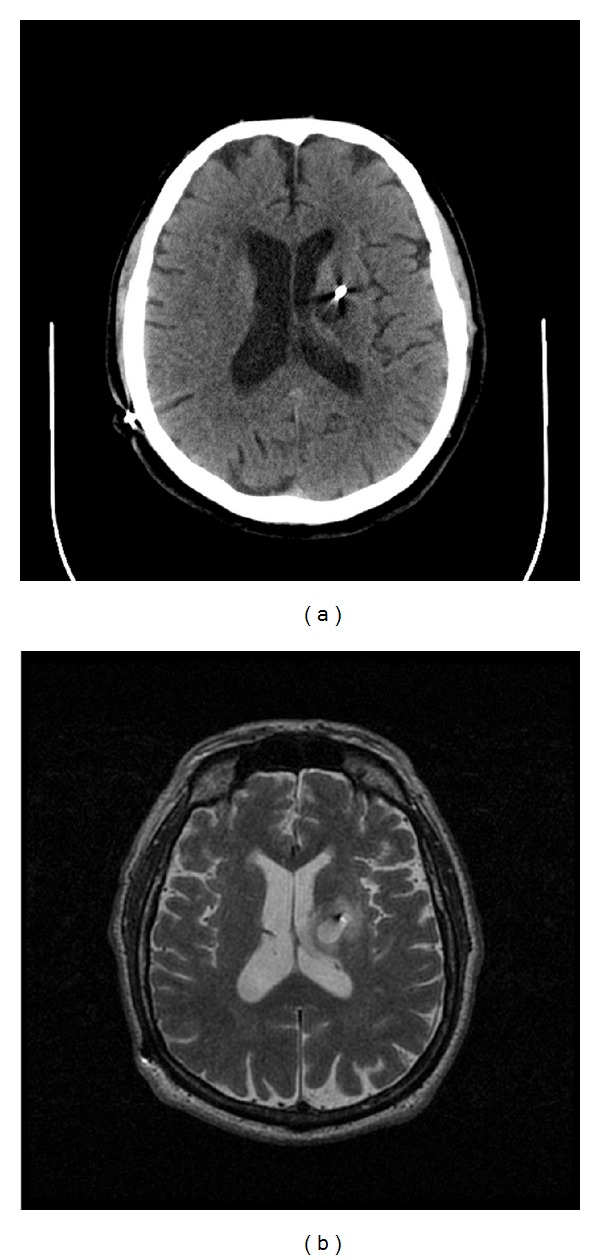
(a) Axial CT without contrast enhancement showing hypodense 1.5 cm ovoid lesion in left basal ganglia surrounding deep brain stimulator lead four months after DBS placement. (b) The lesion appears hyperintense on axial T2-weighted MRI.
